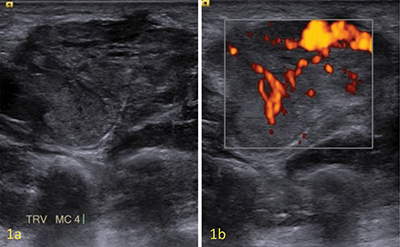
Ultrasound showing a hyopechogenic slightly heterogenous solid soft tissue mass in close contact with the flexor tendons (a), with marked vascularity at Color Doppler (b).
